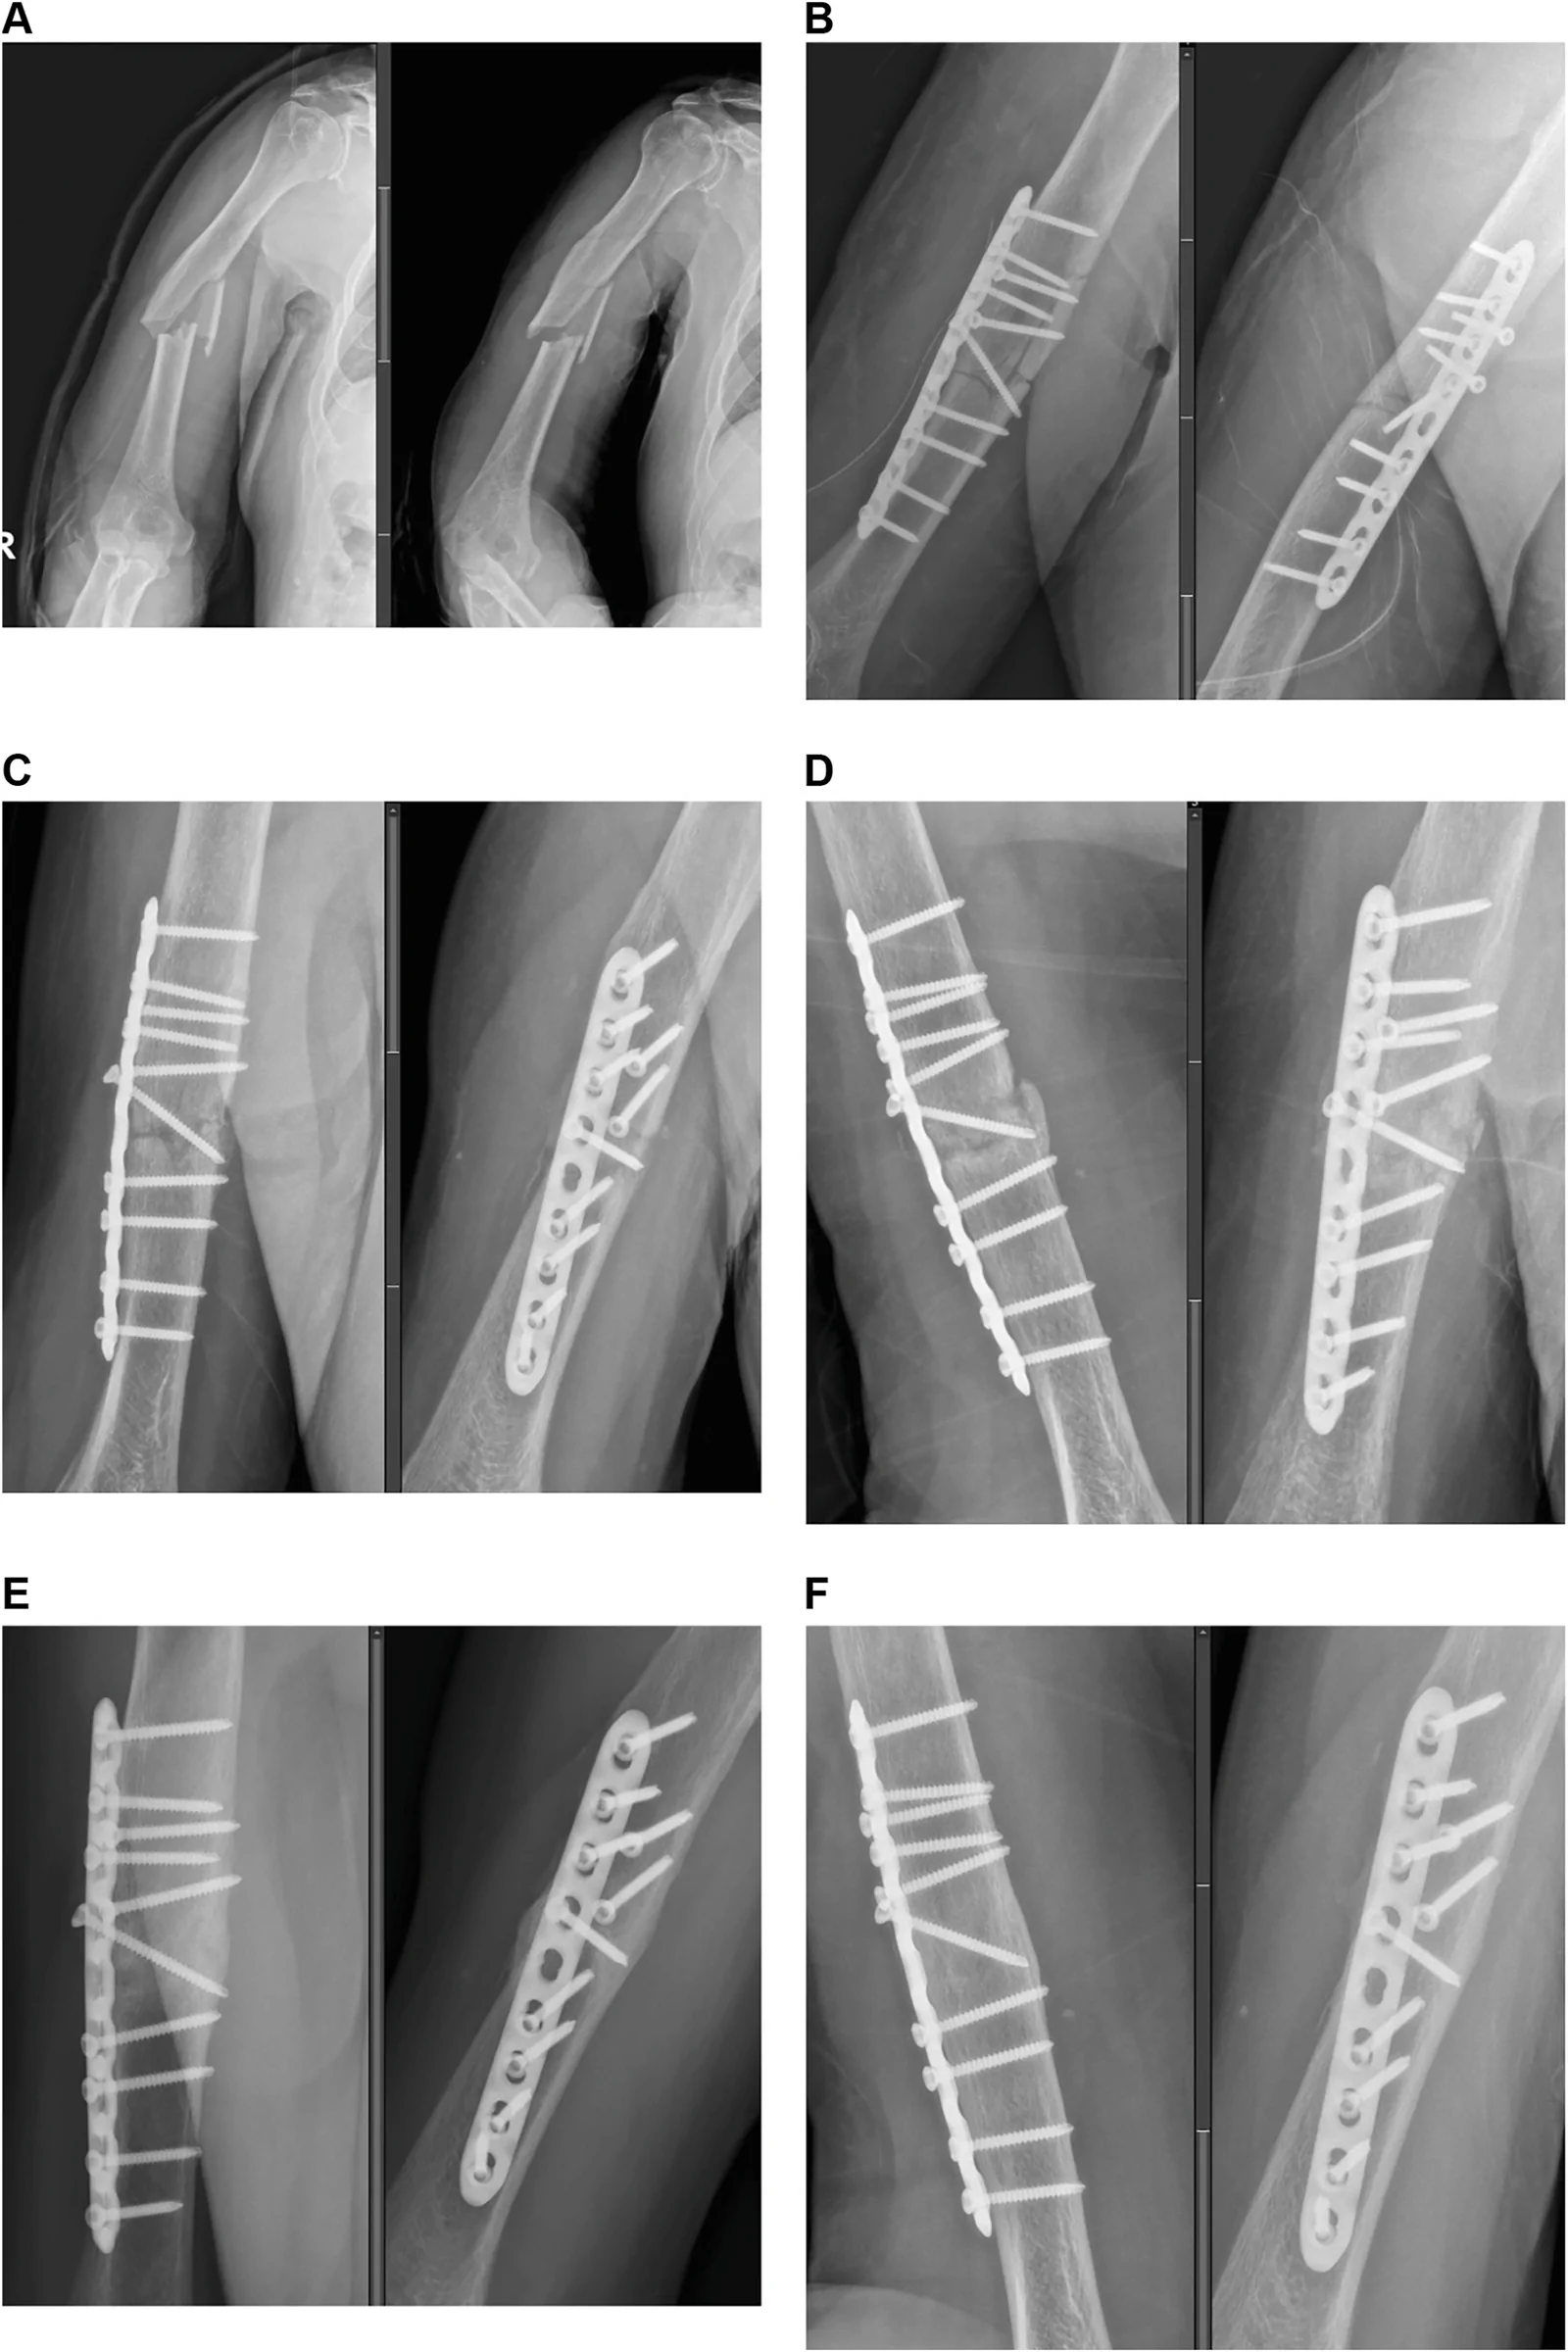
Serial radiographs of Case 3, a 72-year-old man with early delayed union and concern for interfragmentary screw backing-out after plate fixation for a right humeral shaft fracture. (A) Initial fracture radiograph. (B) Immediate post-operative radiograph after plate fixation. (C) Early delayed union at 3.5 months post-operatively with concern for screw backing-out. (D) Radiograph after the second ESWT session showing callus formation. (E) Progressive consolidation at 6 months after the third ESWT session. (F) Maintained union at the 2-year follow-up. *ESWT*, extracorporeal shock wave therapy.
